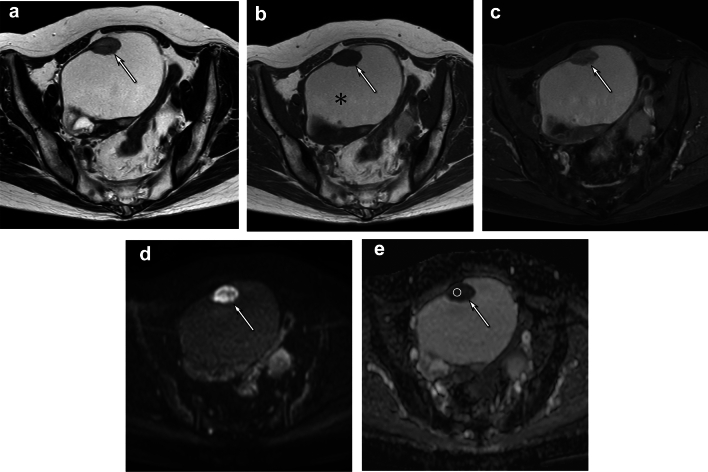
A 66-year-old female with grade 1 OEC. a Axial T2-weighted image showing a cystic mass with solid components (arrow). b Axial T1-weighted image showing a cystic mass with solid components (arrow). The cystic component displays high signal intensity relative to the muscle (asterisk). c Axial fat-suppressed contrast-enhanced T1-weighted image showing homogeneous enhancement of the solid component (arrow). d Axial diffusion-weighted image showing solid component with high signal intensity. e Axial ADC map indicating restricted diffusion (ADC value; 1.11 × 10^−3^ mm.^2^/sec)
